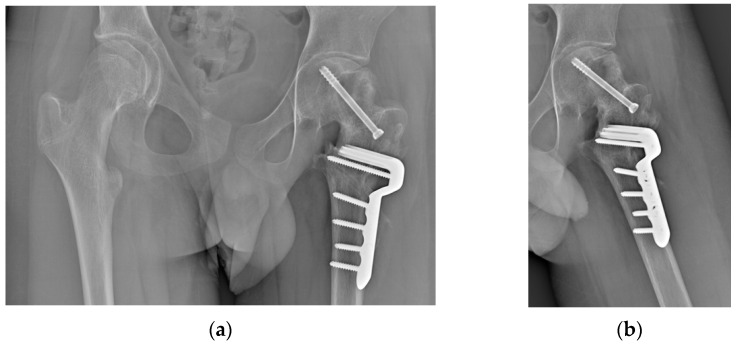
Radiographs at 6 months follow-up. (a) Anteroposterior view; (b) frog-leg view.
